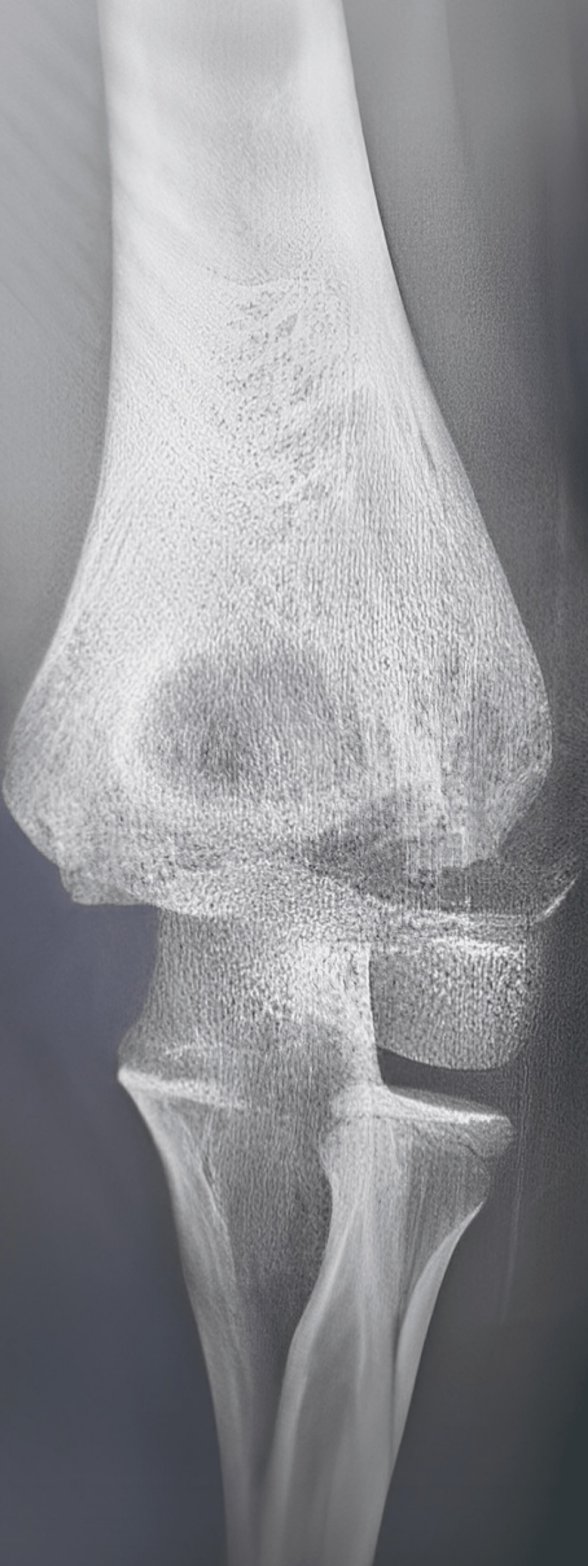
An X-ray was taken of the affected area, which showed a left lateral humeral condyle fracture with displacement
